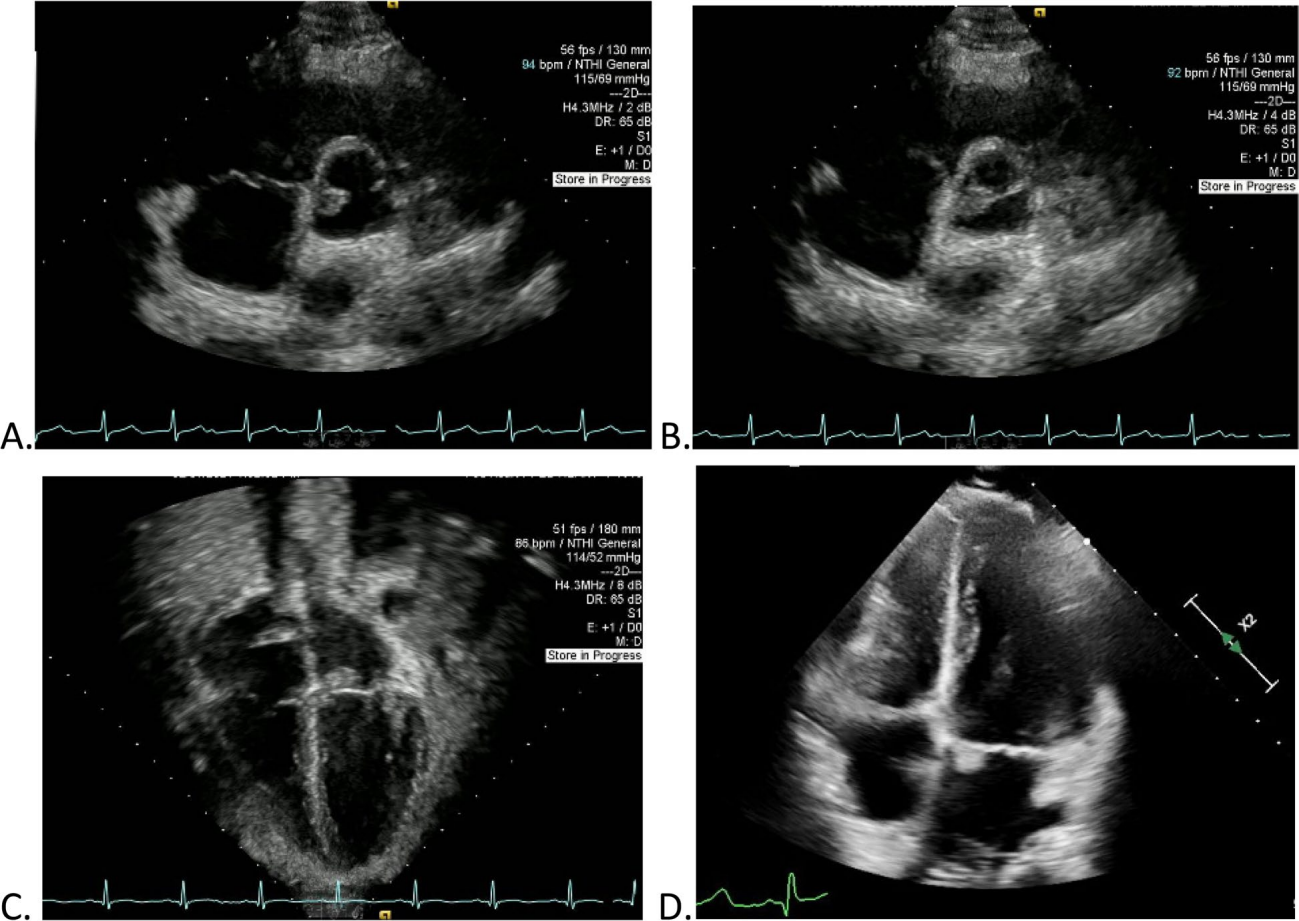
Echocardiogram abnormalities for patient 1 (A, B) and patient 2 (C, D). A, B Demonstration of Aortic Valve Vegetation for Patient 1. C, D Demonstration of Mitral Valve Vegetation for Patient 2
